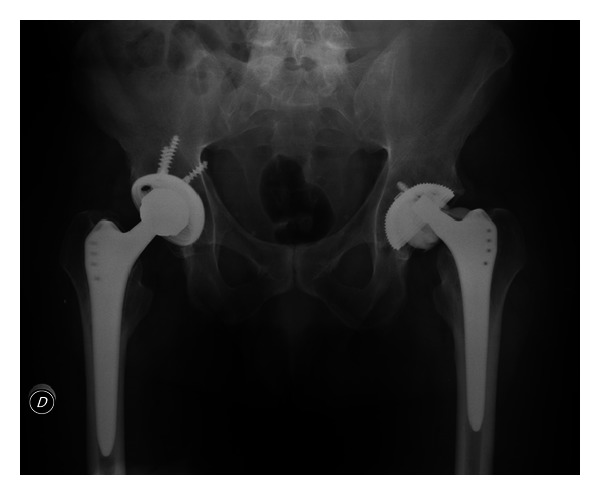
Radiograph showing fracture of the ceramic acetabular insert and the ceramic head.
